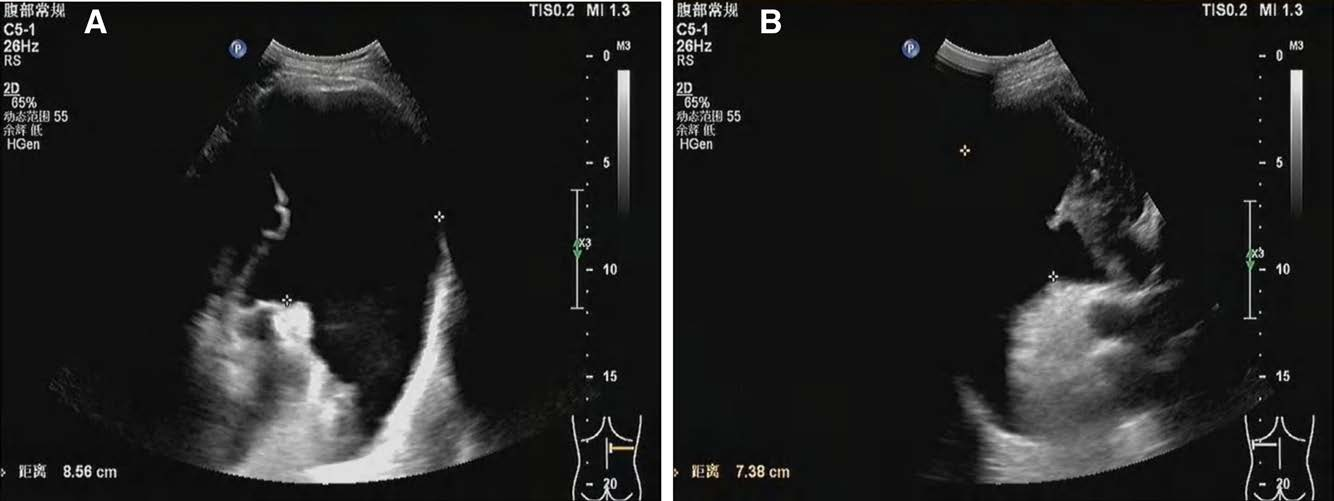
Ultrasound examination revealed bilateral pleural effusion: (A) right pleural cavity: massive effusion with a maximum depth of 8.6 cm (arrow). (B) Left pleural cavity: massive effusion with a maximum depth of 7.4 cm (arrow).
